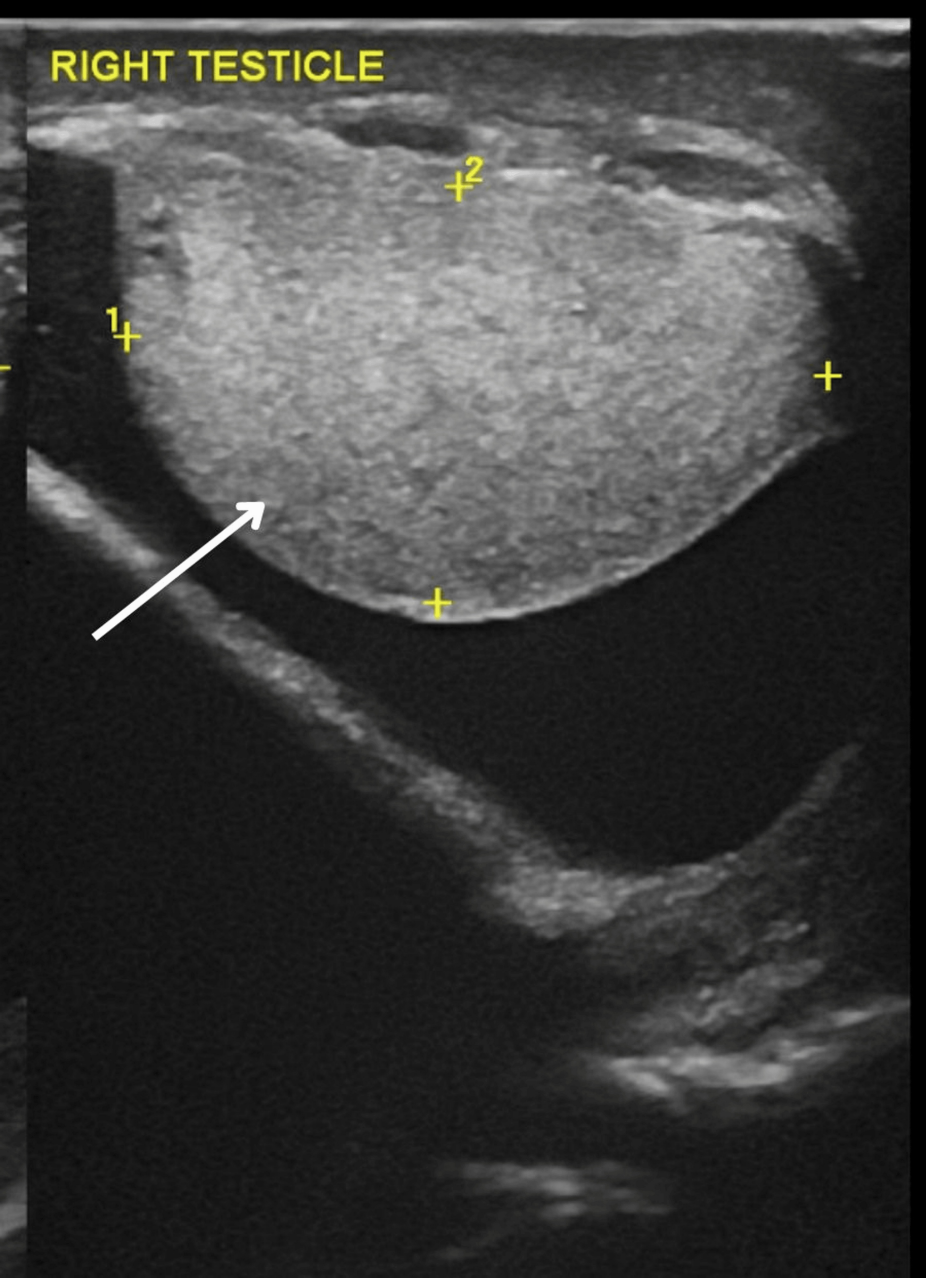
Ultrasound of the scrotum showing the right testis (marked with white arrow)
